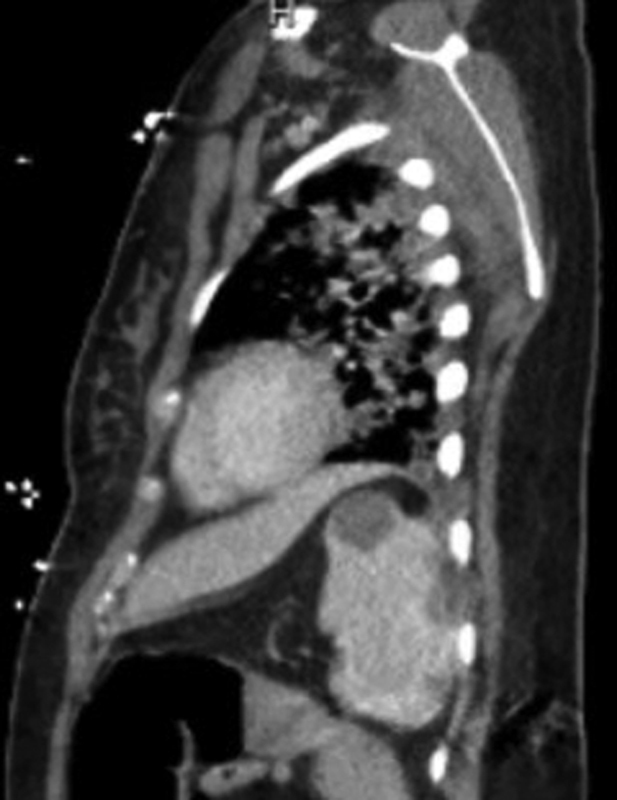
CT abdomen (sagittal view) with multiple infarcts in the spleen. CT, computed tomography.
